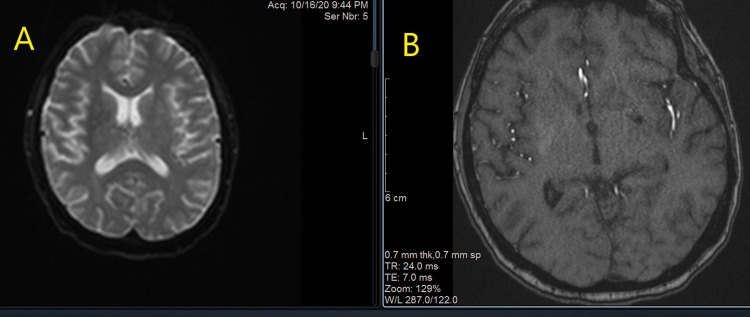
MRI (A) and MRA (B). MRI: magnetic resonance imaging; MRA: magnetic resonance angiography.
